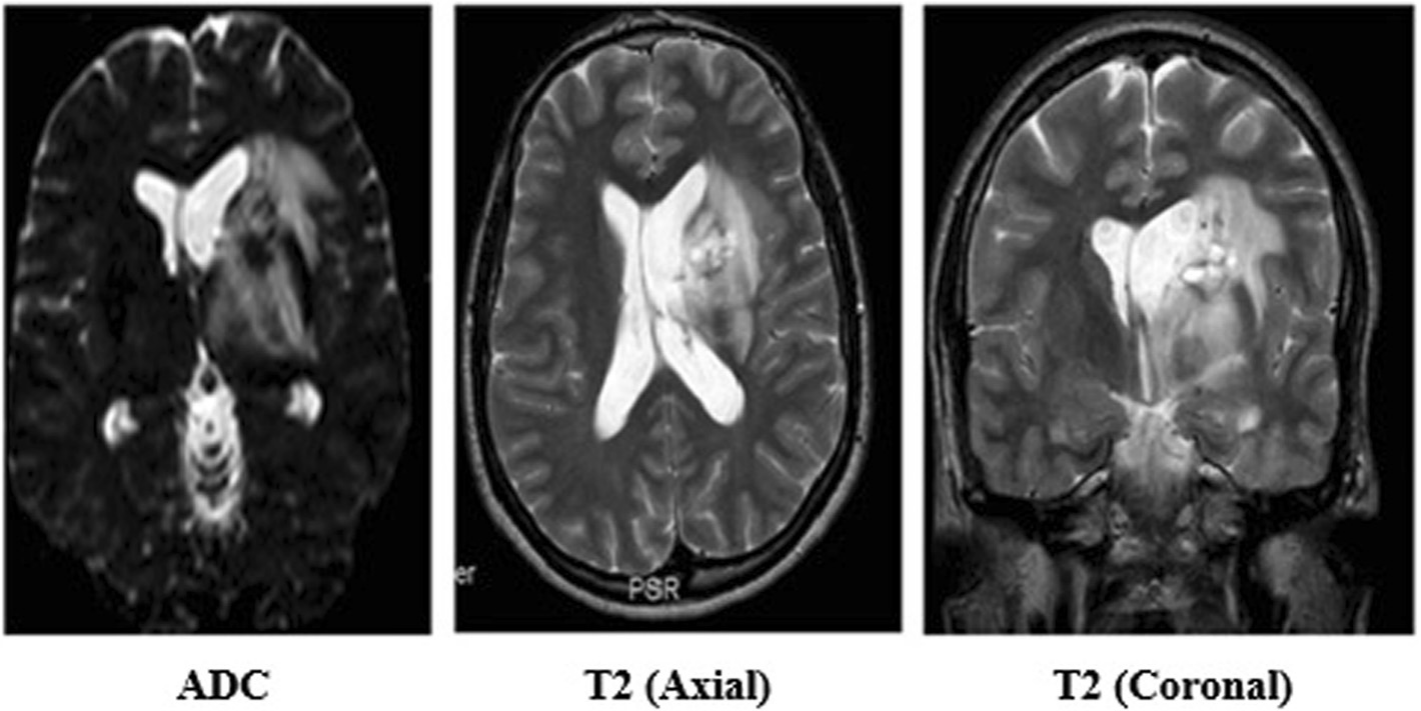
Brain magnetic resonance imaging. Apparent diffusion coefficient (ADC) and T2 images showed altered signal in the left basal ganglia extending to the left thalamus, midbrain and pons with the lesion causing mild fullness of the ipsilateral lateral ventricle due to compression of the left foramen of Monro
